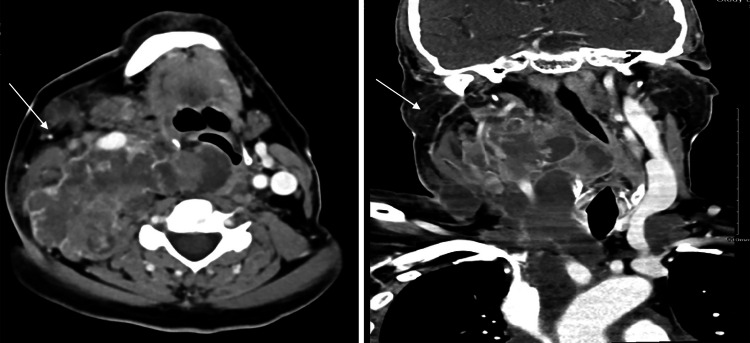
Neck CT axial and coronal views during the emergency room visit following the deterioration of symptoms on lenvatinib treatment and anaplastic transformation The images show further progression in size and mass effect with airway deviation CT: computed tomography
